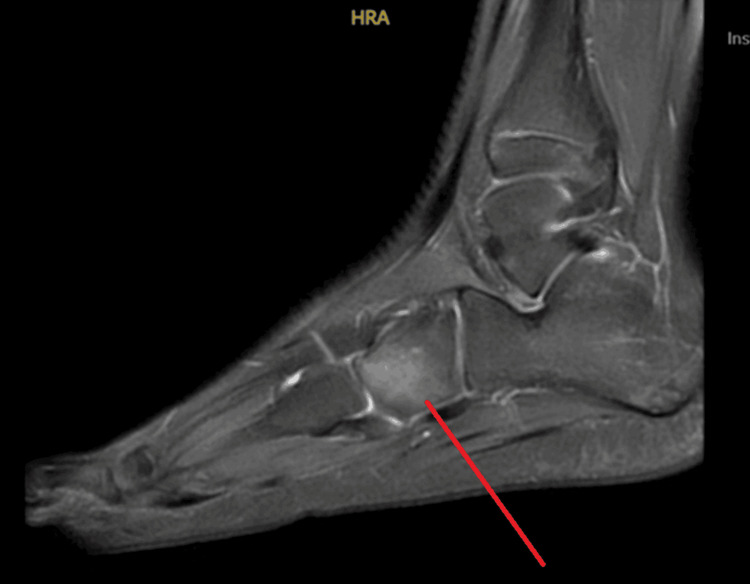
Sagittal MRI of the Right Foot Sagittal MRI demonstrates bone marrow edema in the cuboid.
